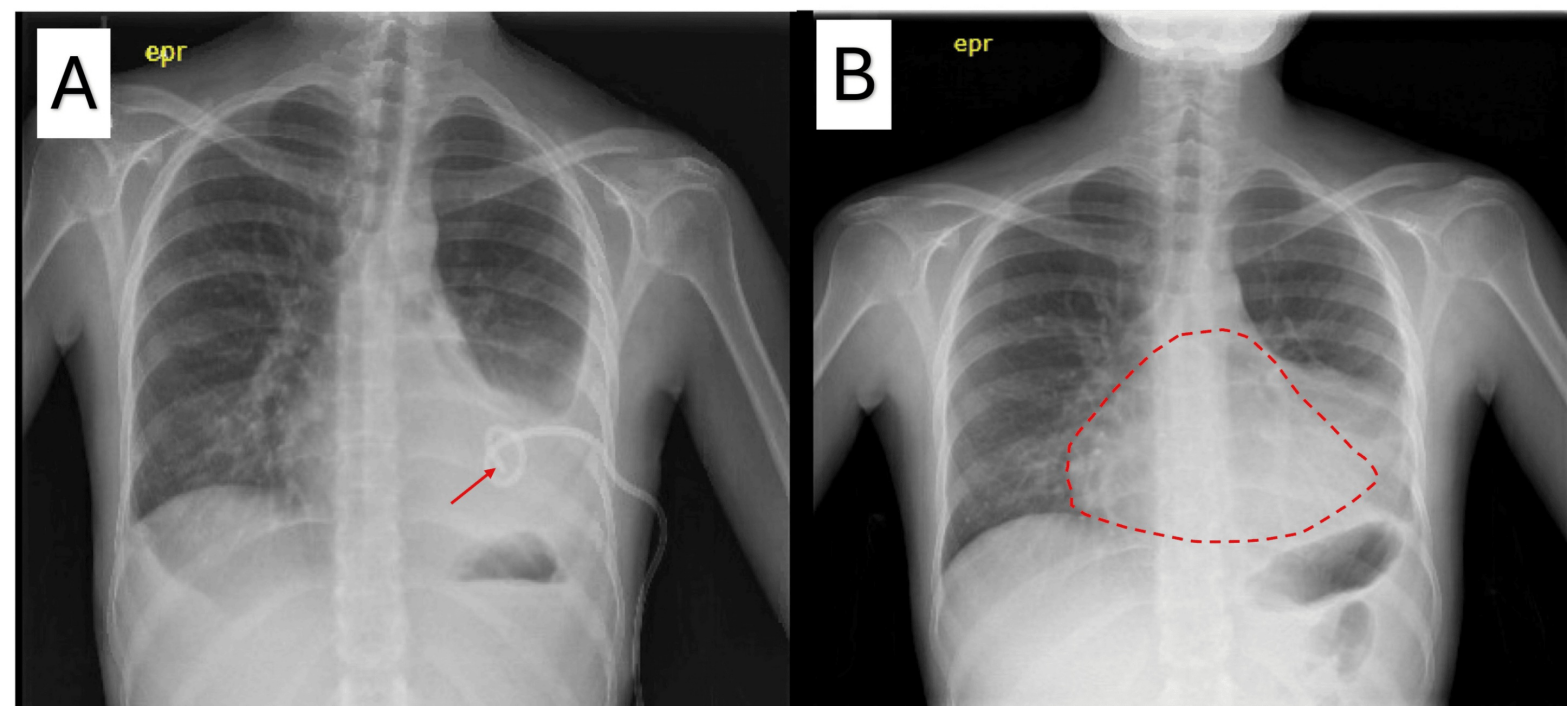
(A) Posterior-anterior chest radiograph showing partial resolution of left-sided pleural effusion with chest drain insertion. The red arrow points towards the correctly sited chest tube (left) and (B) repeat chest radiograph two months later prompting concern for a pericardial effusion with the red dotted line highlighting cardiomegaly (right).
